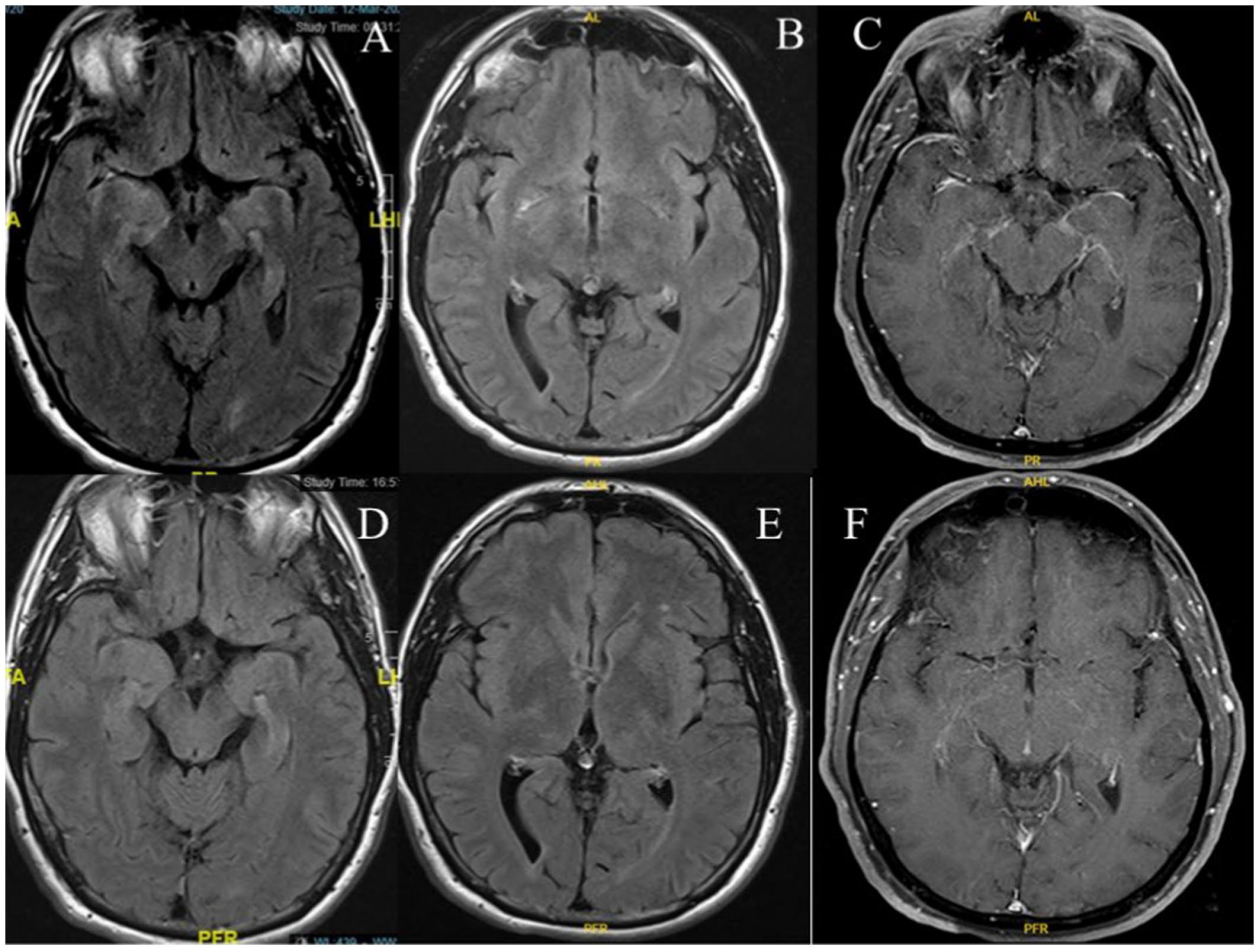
Brain MRI shows a T2 hyperintense lesion in the mesial temporal lobes (A), right sub-lenticular region on axial FLAIR (B), and gadolinium enhancement in the right>left leptomeningeal, perisylvian regions, and mesial temporal lobes on axial T1-weighted post-gadolinium administration (C). Follow-up MRI shows resolution of findings on axial FLAIR (D,E), and T1-weighted post-gadolinium administration (F).
